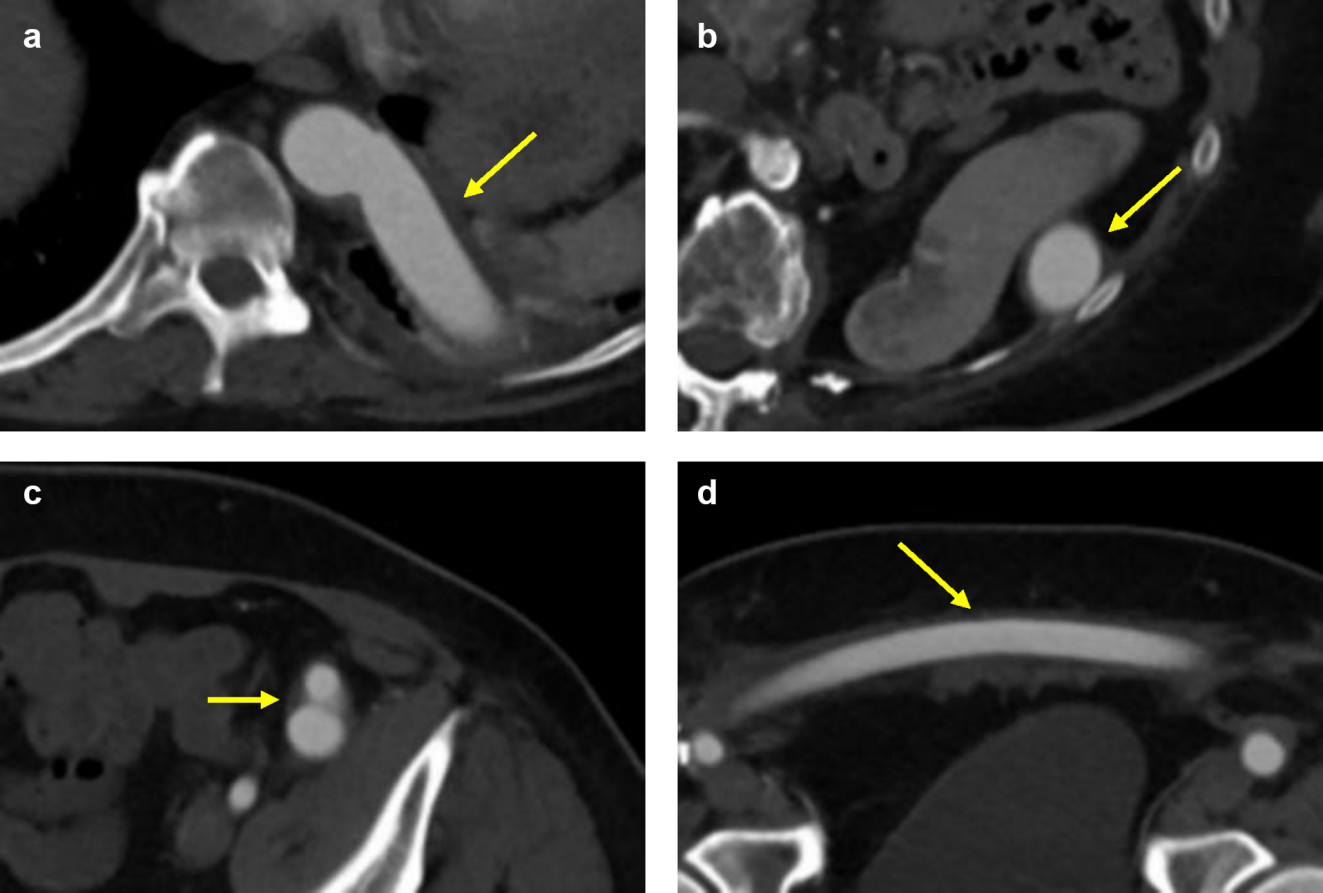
Axial computed tomography angiography (CTA) demonstrating the graft (yellow arrow) as it traverses the diaphragm and enters the retroperitoneal space (a), passes deep to the spleen (b), reaches the level of graft bifurcation (c), and courses subcutaneously toward the right groin (d).
